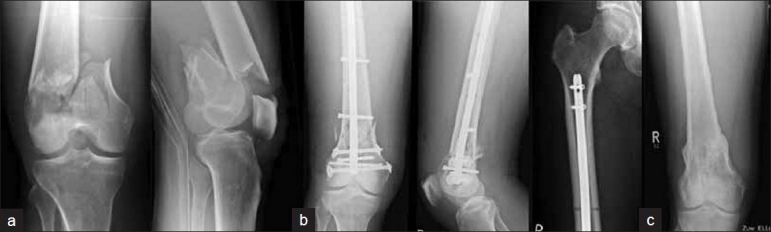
(a) Anteroposterior and lateral radiographs of right knee of a 65-year-old male who suffered a C1 distal femur fracture. (b) The fracture was stabilized using SCN retrograde nail. (c) Radiographs of knee following removal of nail depicting sound bony union and consolidation at fracture site
